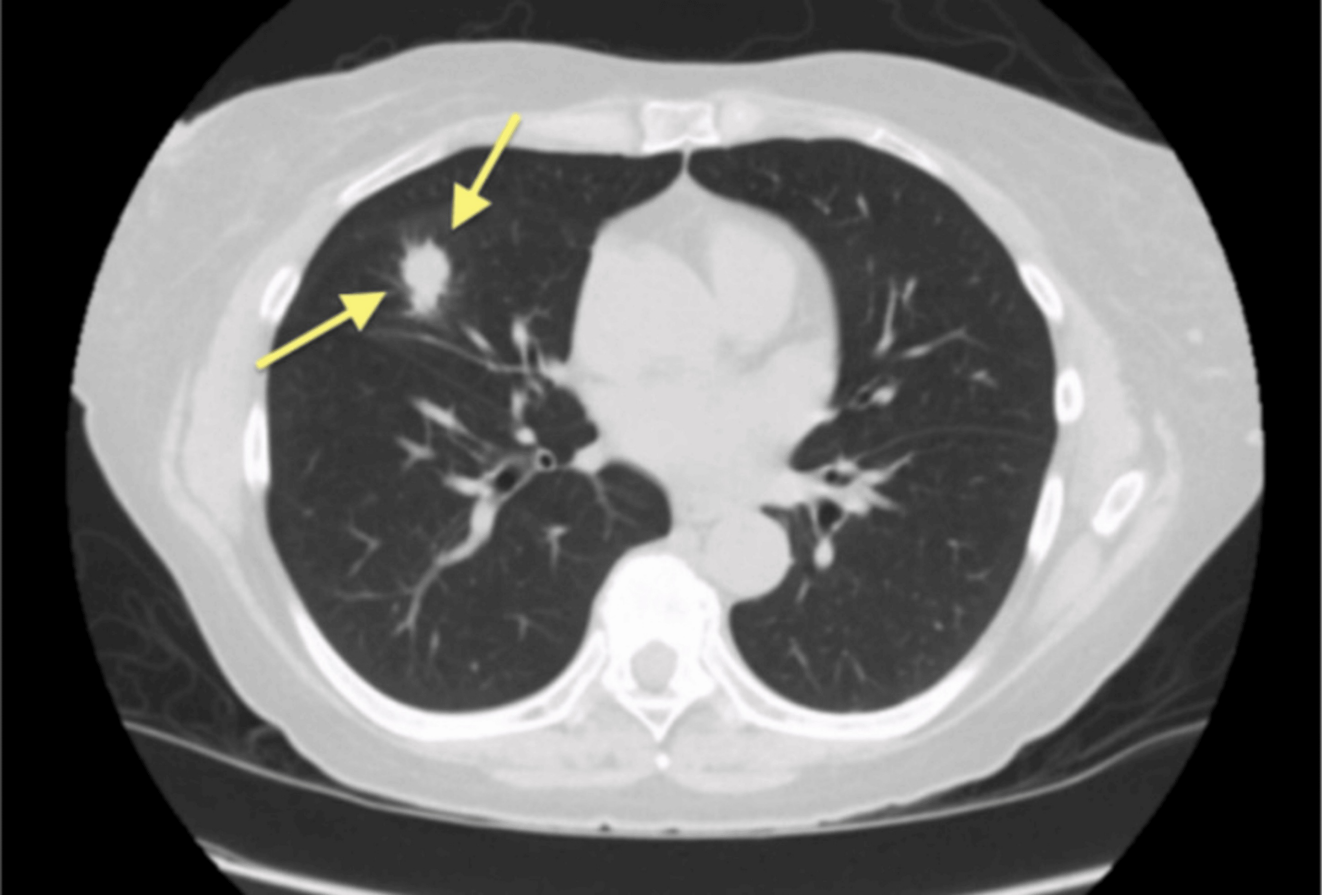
CT of the chest showing a nodule in the right middle lobe. CT, computed tomography
